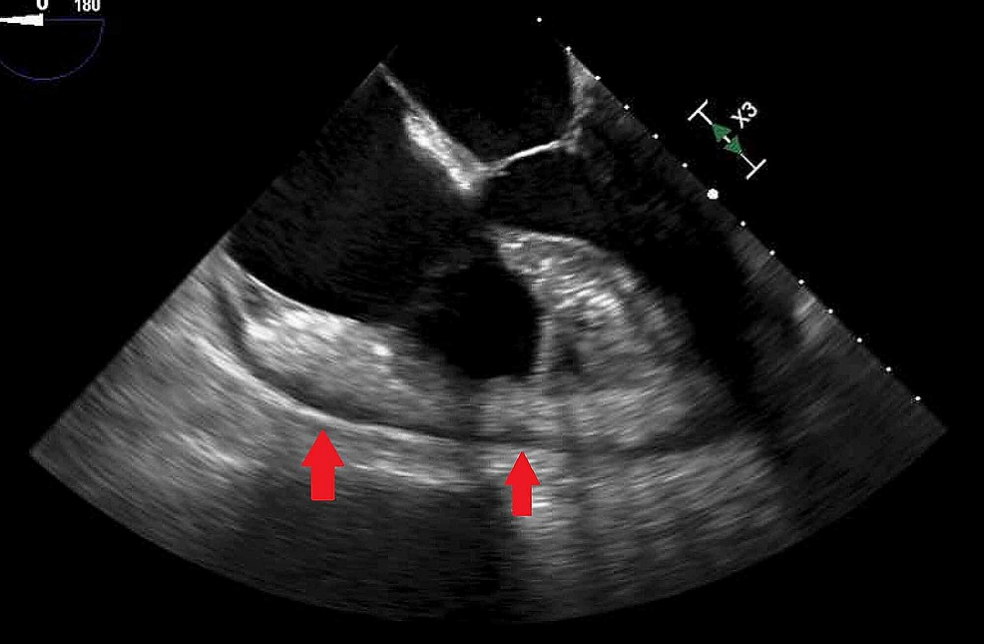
Echocardiogram image performed after pericardial fluid drainage showing small residual effusion (arrows).
